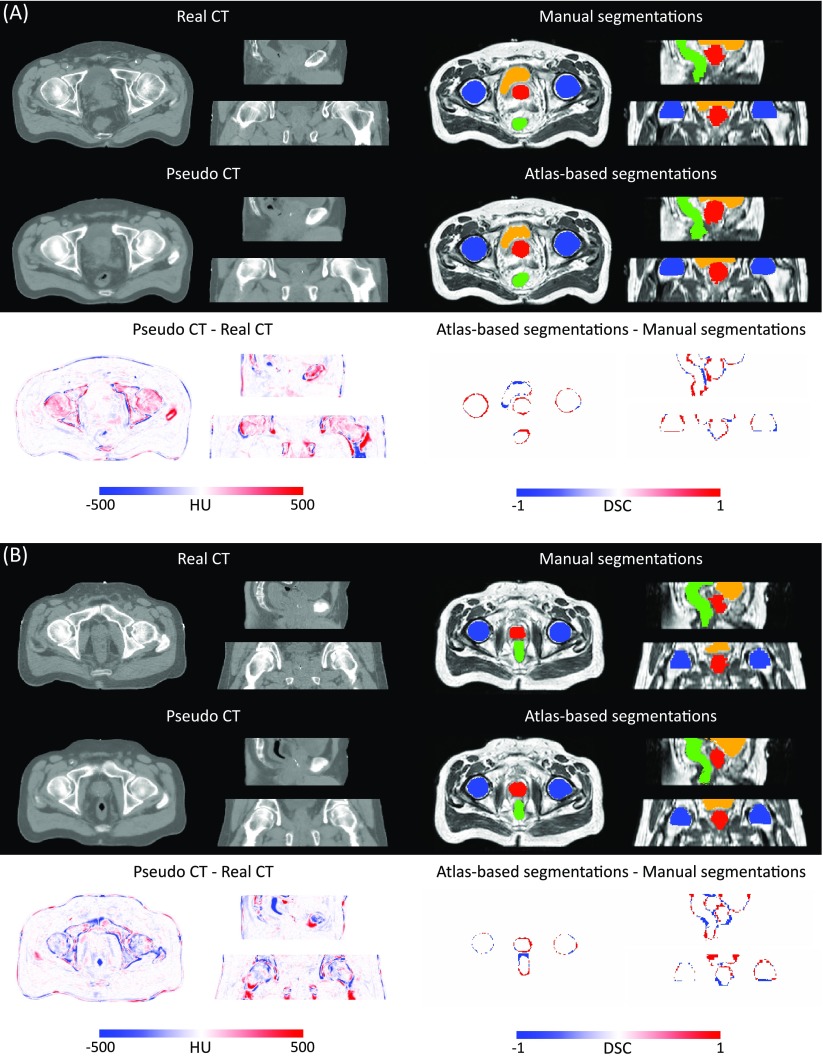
Categorical segmentations of the prostate (red), bladder (orange), rectum (green) and femur heads (blue), pseudo CT obtained from the T1-weighted and T2-weighted MR images of the patient after four iterations of the proposed joint iterative segmentation and image synthesis framework, and difference images for subjects with the best (top) and worst (bottom) results.
